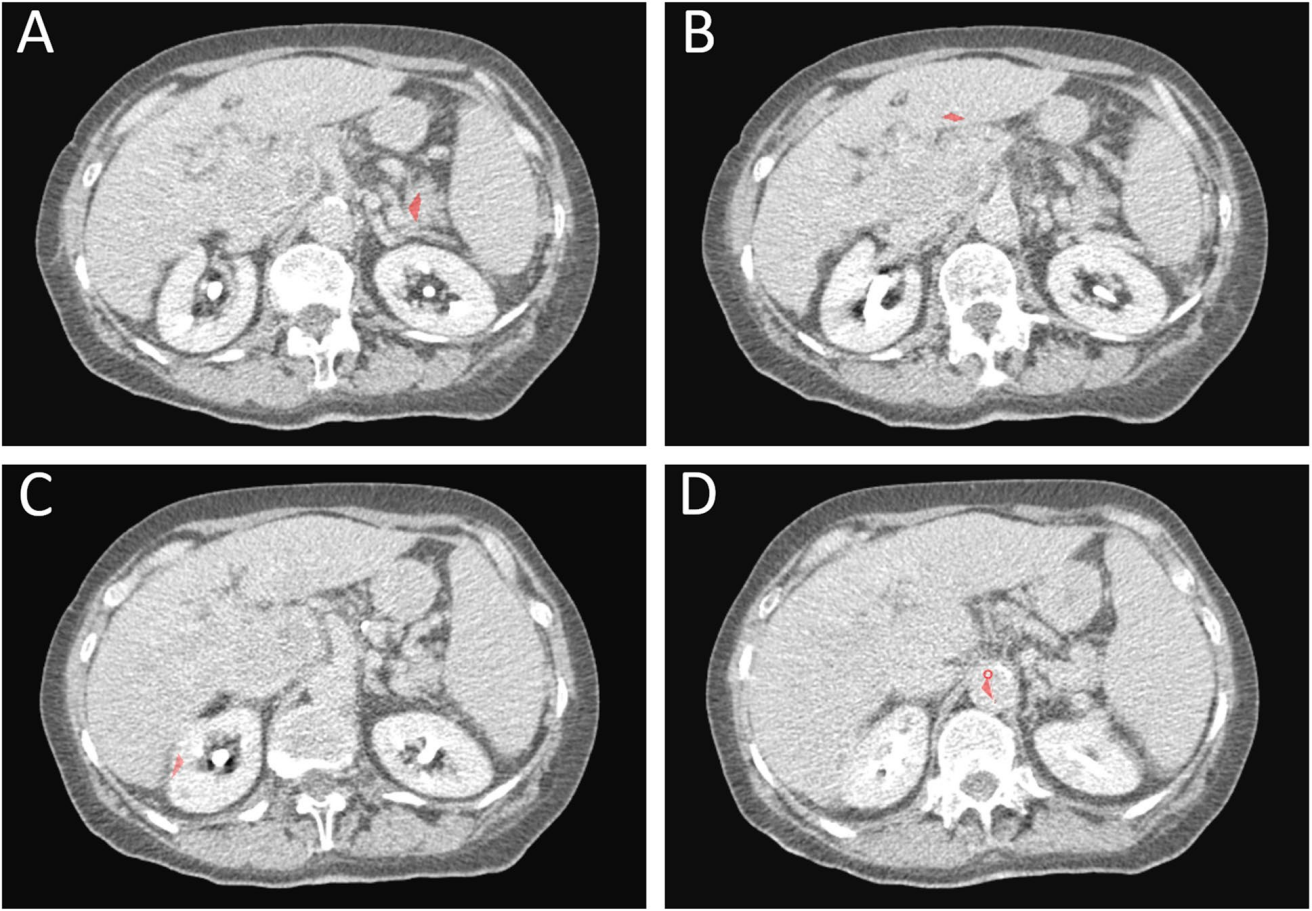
ROIs drawn to compute the time-enhancement curves. In A, a ROI on the pancreas was drawn on the largest pancreatic portion included in the CT study volume. While this portion is usually represented by the pancreatic head, in this case the pancreatic head was not included in the study volume due to a pathologic lymph node and the ROI was drawn on the pancreatic tail. B ROI drawn on an HCC focus. C ROI drawn on the cortex of the right kidney. D ROI drawn in the aorta, between the emergency of the superior mesenteric artery and of the renal arteries
